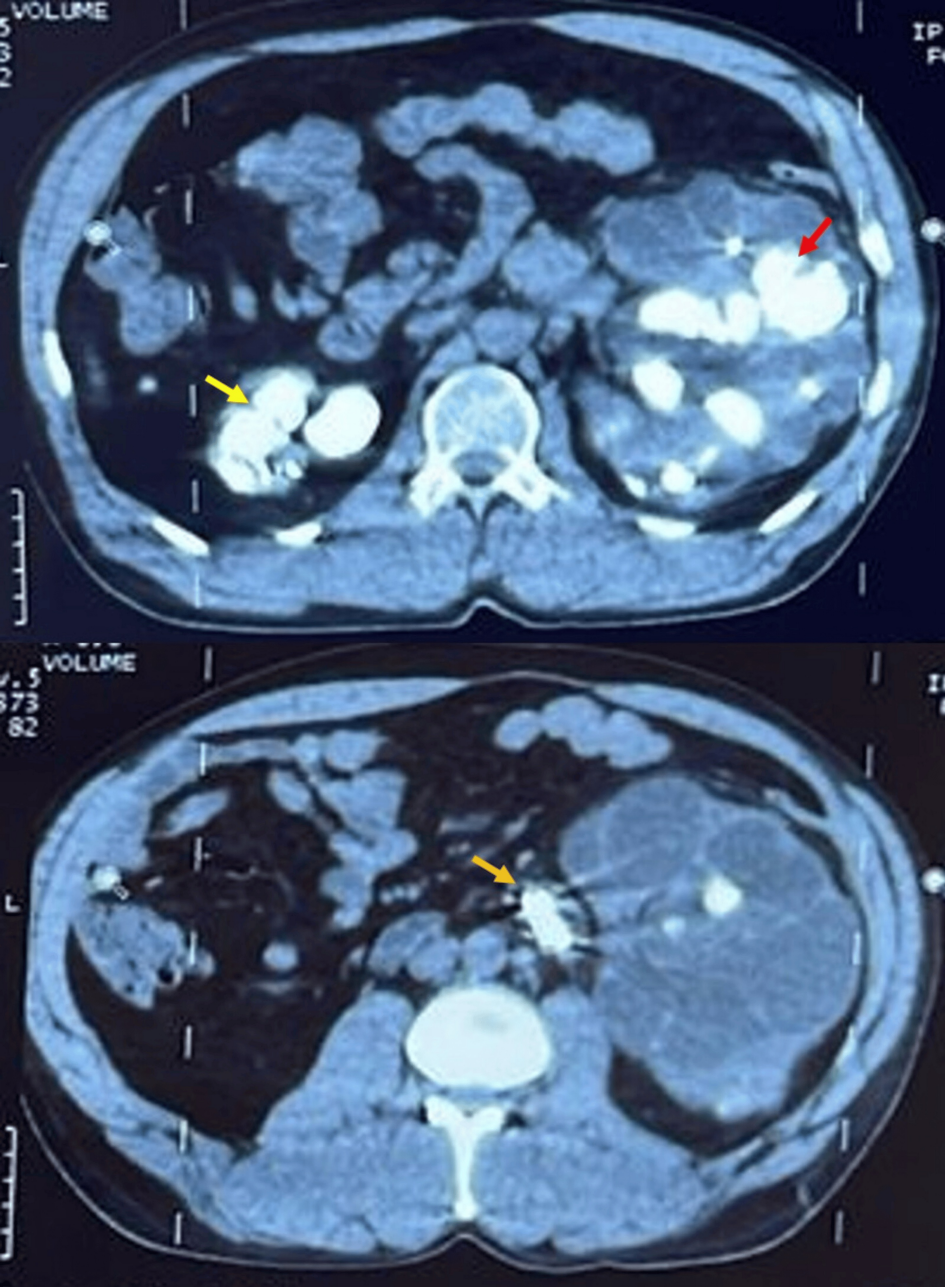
Non-contrast axial CT scan showing a small right kidney with multiple spontaneous hyperdensities corresponding to renal calculi (yellow arrow). The left kidney is hypertrophied as a compensatory mechanism and also presents multiple spontaneous hyperdensities (red arrow). A left ureteral calculus (orange arrow) is visible along the two double-J stents.
